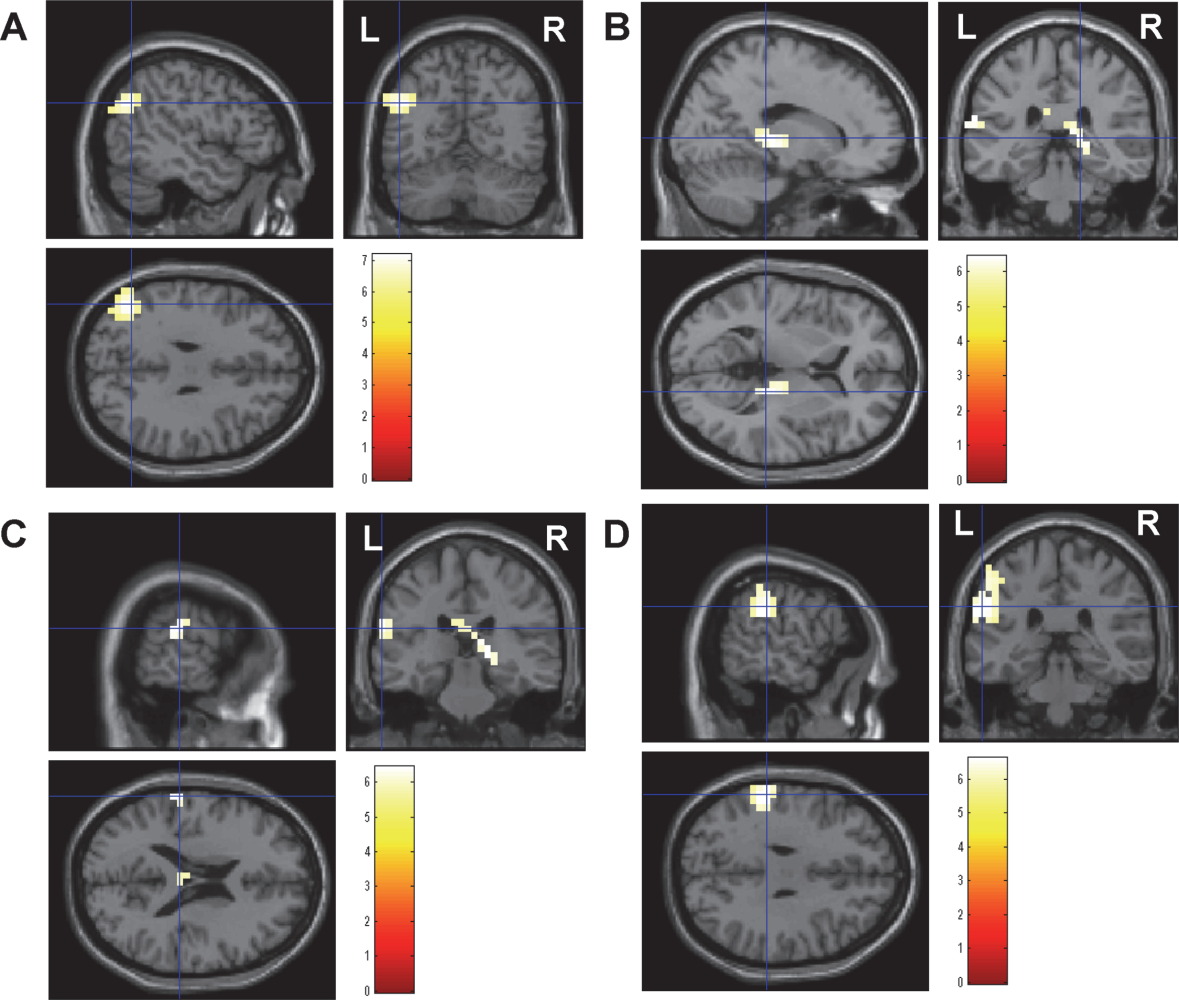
Statistical parametric maps of brain regions where power was lower in the fatigue experiment than in the non-fatigue experiment: the delta band (1–4 Hz) power in the left BA 39 (A), alpha band (8–13 Hz) power in the right pulvinar nucleus (B) and the left Brodmann’s area (BA) 40 (C), and beta band (13–25 Hz) power in the left BA 40 (D). Statistical parametric maps are superimposed on surface-rendered high-resolution magnetic resonance images. The right (R) and left (L) sides are indicated. The color bars indicate t-values. Random-effect analyses of 13 participants, *P* < 0.05, familywise-error corrected for the entire search volumes.
